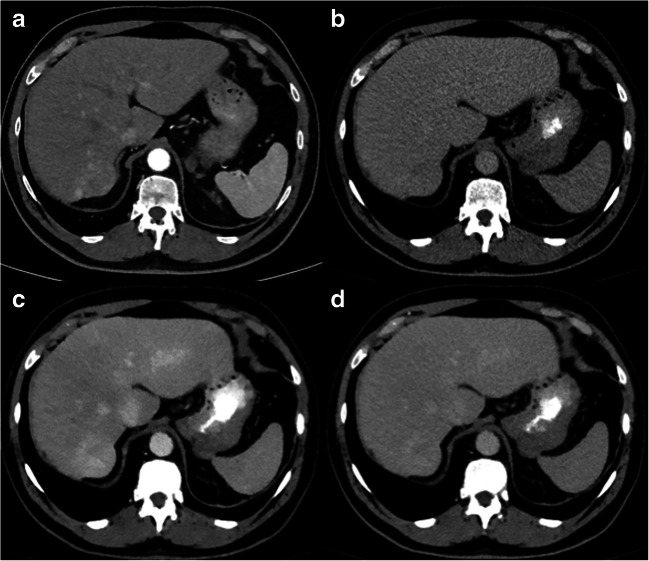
Axial CT images showing an arterially hyper-enhancing heterogeneous lesion in liver segment VII (a) with washout in delayed phase on CIs (b) and suggested on 60-keV images (d). However, the lesion partially exhibited hyperdense Hounsfield units on 40-keV images compared to the liver parenchyma (c). The lesion also showed washout in the reference MRI and was categorized as LiRADS 5, which was proved by biopsy
